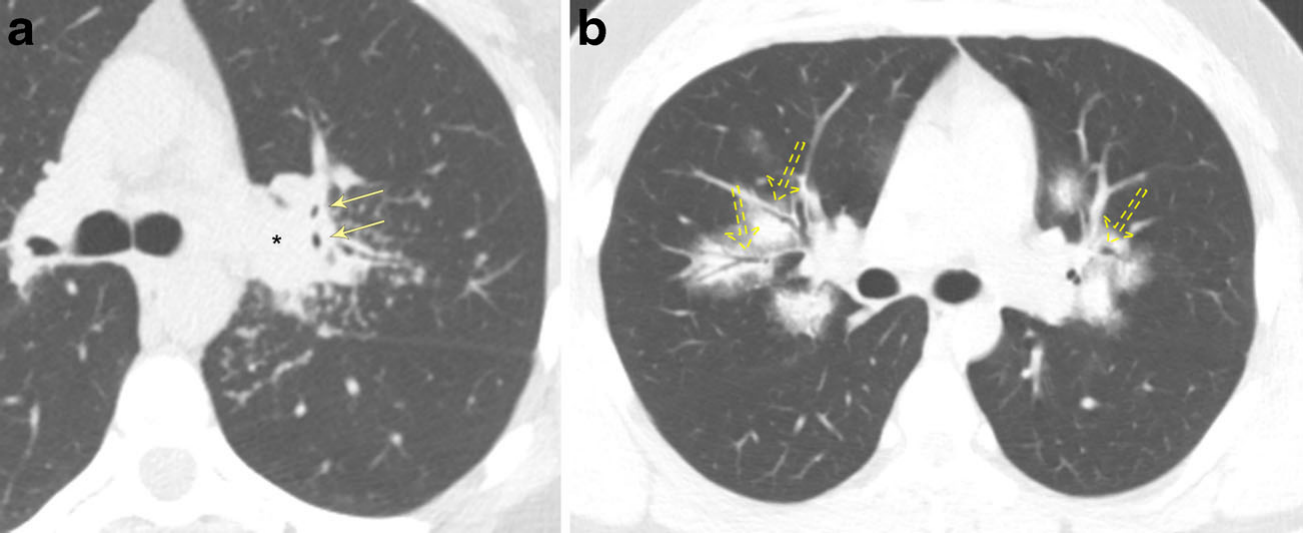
Bronchial obstruction due to granulomatous disease. a Axial CT image of sarcoidosis demonstrating perihilar lymphadenopathy (*) and pulmonary granulomas causing extrinsic compression of several left upper lobe segmental bronchi (*arrows*). b Axial CT imaging of granulomatosis with polyangitis demonstrates infiltrative changes of the pulmonary parenchyma in a perihilar/bronchovascular distribution causing narrowing of the associated bronchi in a bilateral upper lobe predominance with sparing of the apices. The associated ground-glass opacities suggest the presence of haemorrhage
